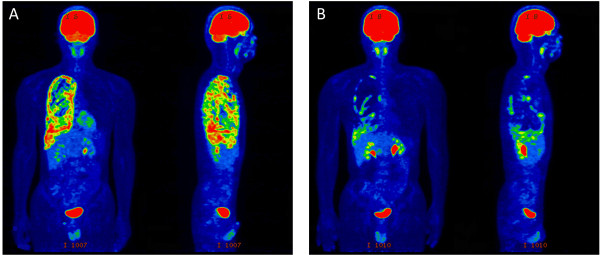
FDG PET image. A: Multiple lesions of intense FDG uptake in the right pleura at presentation. B: Clearly decreased positive signals after 6 months of antituberculous chemotherapy.
